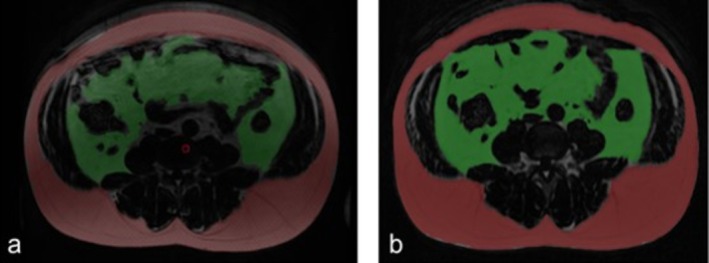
Magnetic resonance imaging showing the areas of visceral adipose tissue and subcutaneous adipose tissue.
